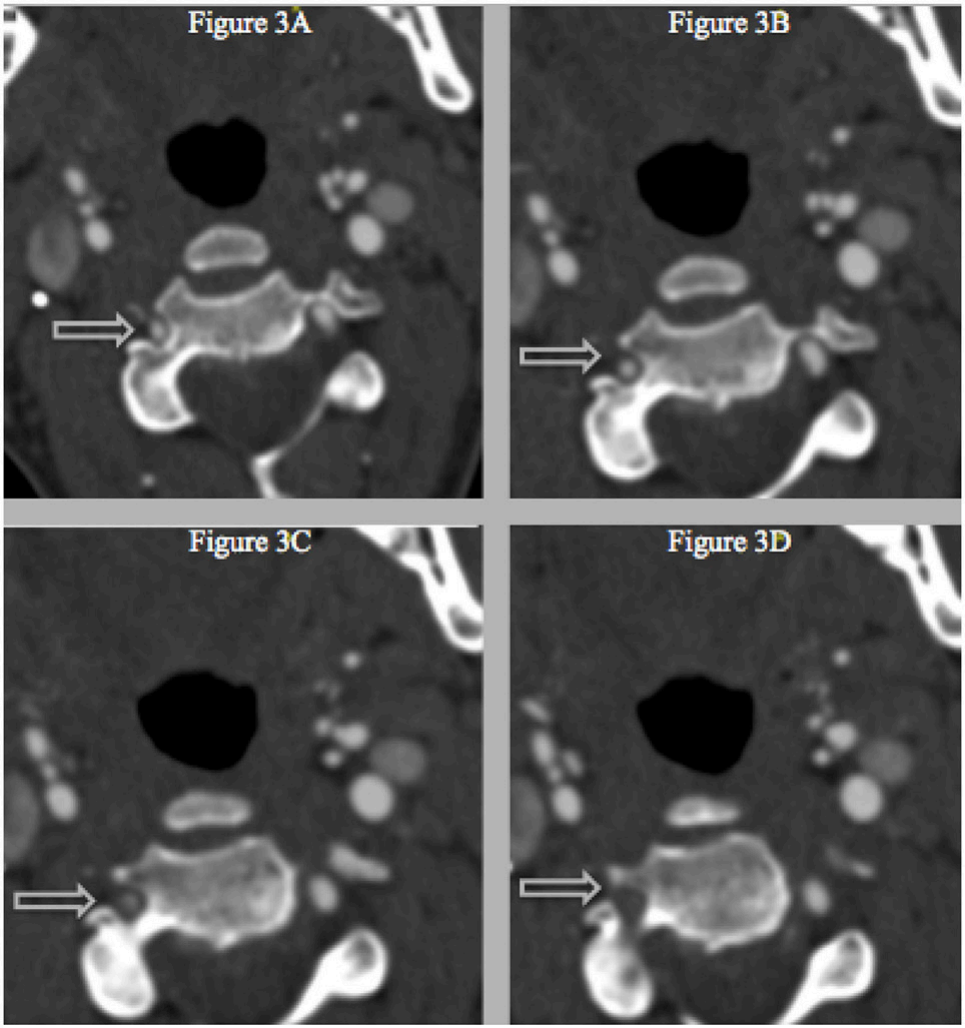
(A–D) Successive CT with IV-contrast images (0.75 mm) depicting a segment of non-opacification within the right vertebral artery and a normal vertebral canal at level C3, which suggests that the stenosis is not related to vascular hypoplasia within that area.
